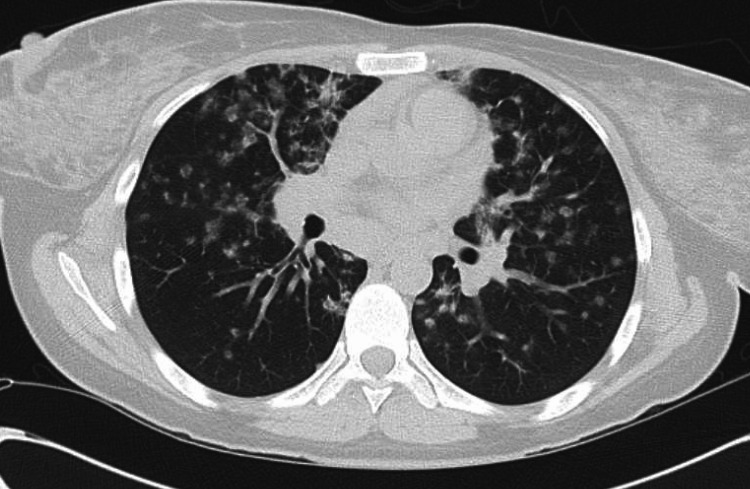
The patients initial chest computed tomography showing numerous pulmonary nodules with ground-glass appearance along with bilateral hilar lymphadenopathies.
